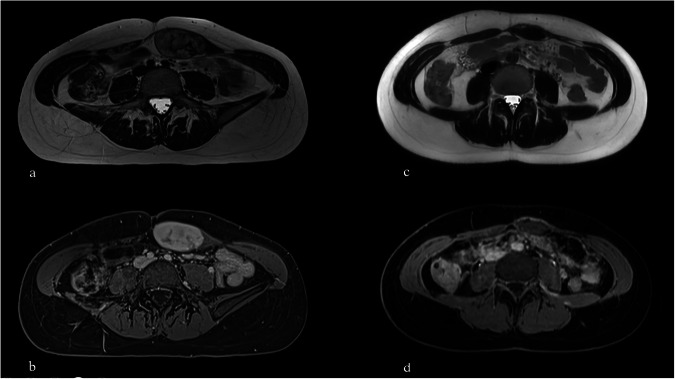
Complete response according to M-RECIST. a T2-weighted and (b) contrast-enhanced T1-weighted images of a DF arising in the anterior abdominal wall at baseline; c T2-weighted and (d) contrast-enhanced T1-weighted images after PC. The patient was classified as stable disease (SD) according to RECIST 1.1 due to subthreshold variation of the tumor’s longest diameter. The case was classified as complete response (CR) with M-RECIST due to diffuse fibrotic involution of the lesion, depicted by a marked decrease in T2 signal, and no evidence of nodular/mass-like enhancement after intravenous administration of gadolinium-based contrast agents; faint late enhancement of a perilesional fibrotic capsule, consistent with a CR, was also demonstrated
